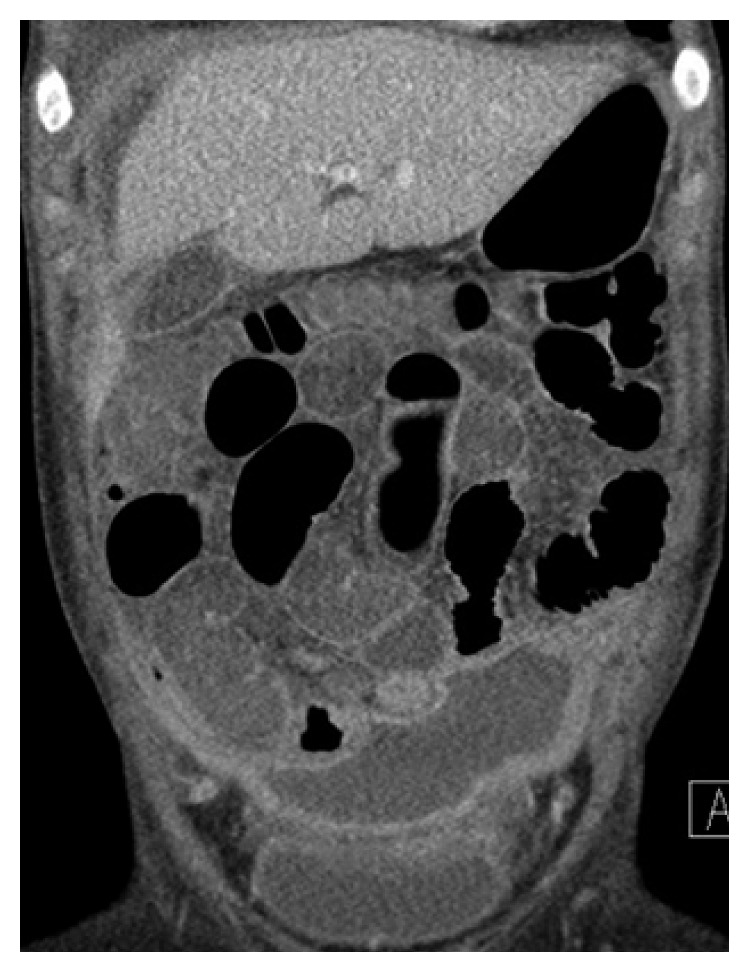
CT scan nine days after surgery with persistent ascites and peritoneal enhancement.
